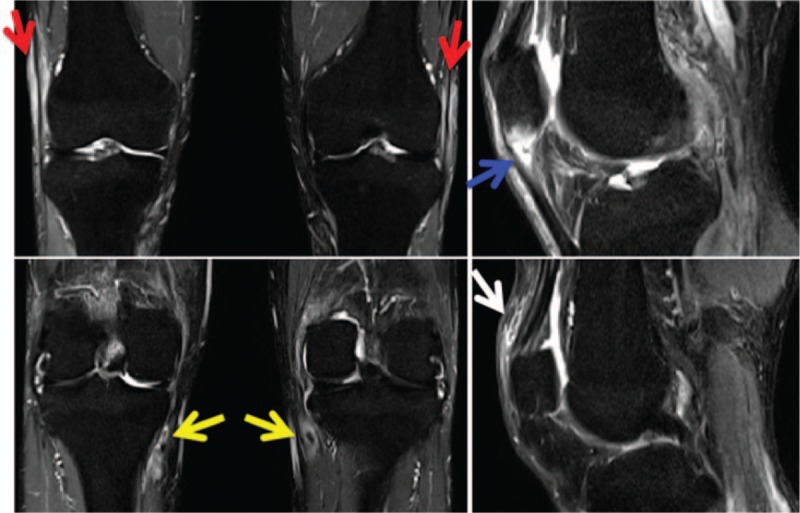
MRI of the bilateral knees, showing ligamentitis of the bilateral iliotibial tract (red arrow), bilateral pes anserine tendinitis (yellow arrow), enthesitis of the right proximal patellar ligament on the inferior pole of the right patella (blue arrow), and enthesitis of the left quadricep tendon on the superior pole of the left patella (white arrow).
